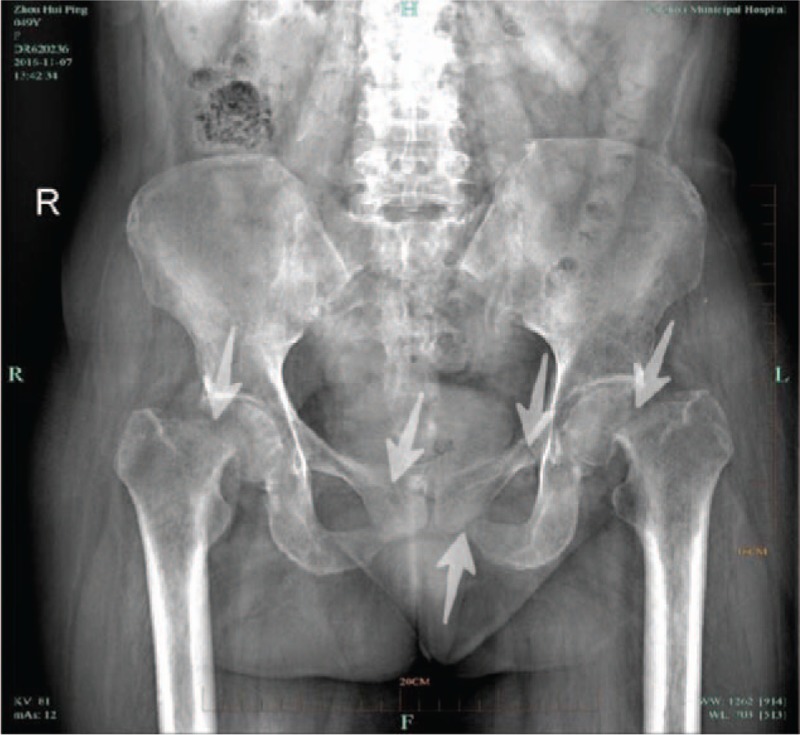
Preoperative pelvic X-ray suggested that bilateral femoral neck fracture and bilateral pubic fractures (indicated by white arrow).
